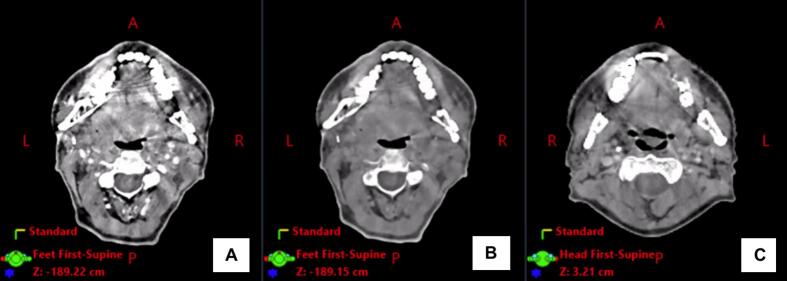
Head and neck images acquired with different CT techniques. From left to right: EID-CT, PCD-CT 50 keV, PCD-CT 140 keV.
